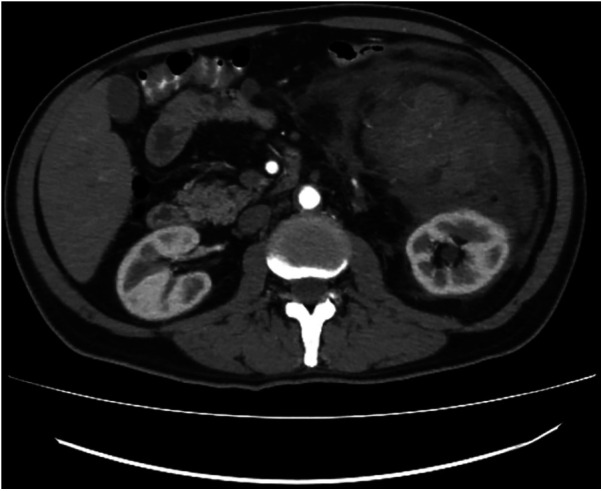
Ct angio, CT findings for case 2.
